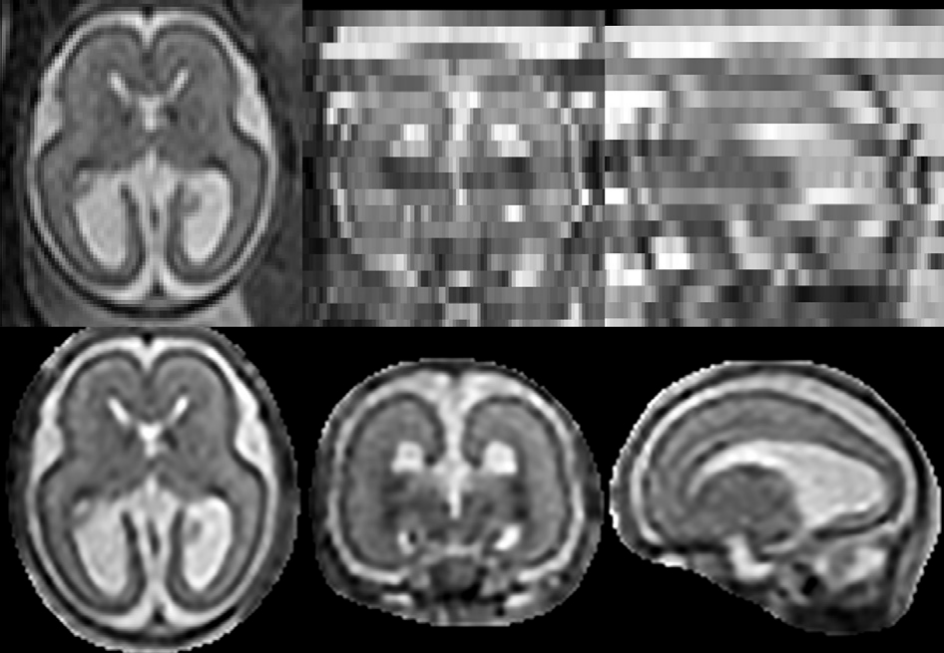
Reconstructed fetal brain MRI of a subject with GA 23 weeks using only five stacks of slices. First row: One of the acquired stacks. Second row: Reconstructed volume.
